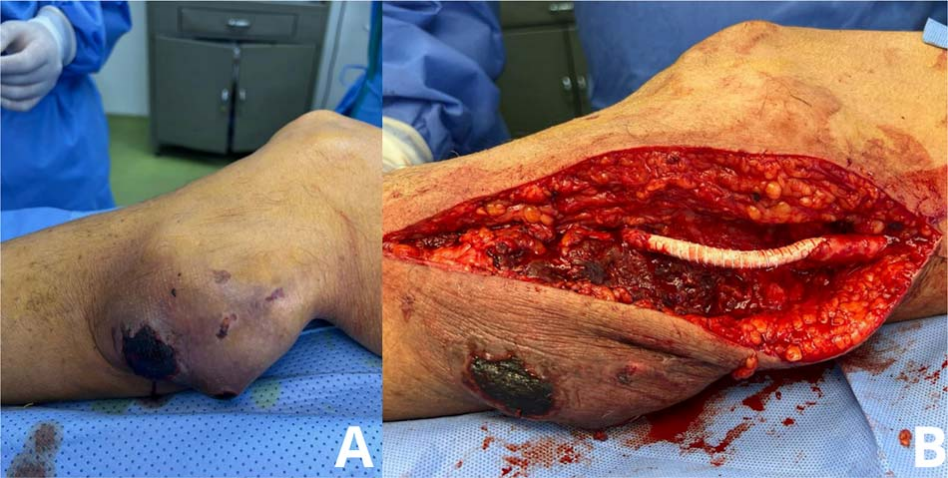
(A, B) CTA scans of the lower extremities reveal a pseudoaneurysm in the right popliteal artery, indicating vascular injury with disrupted arterial flow. (C) Angiographic study shows the pseudoaneurysm sac and confirms a tear in the popliteal artery, with no evidence of distal thrombus or embolization.
